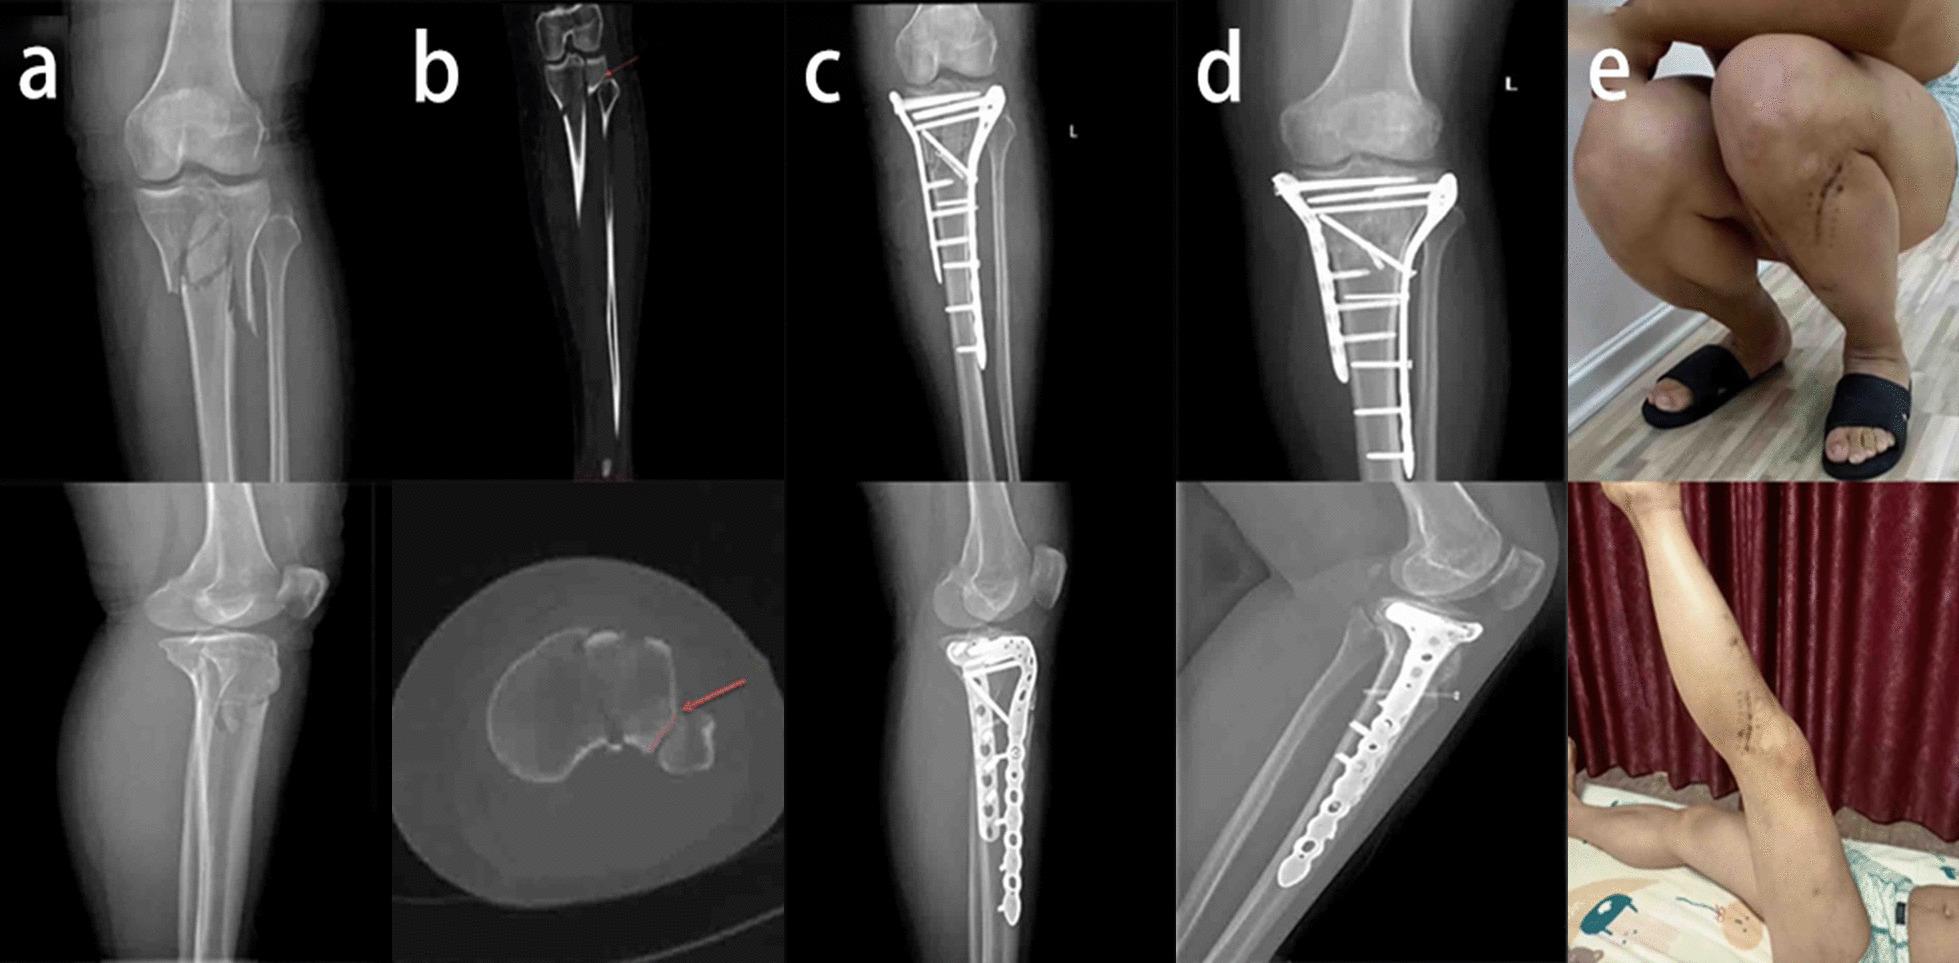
a Preoperative anteroposterior and lateral radiographs of a 45-year-old male patient. b CT scan showing that the proximal fibula and PJF (red arrow) were intact. c Postoperative anteroposterior and lateral radiographs showing fixation of the TPFs with double plates. d Anteroposterior and lateral radiographs indicating good fracture healing one year after surgery. e Satisfactory knee motor function and lower limb muscle strength
